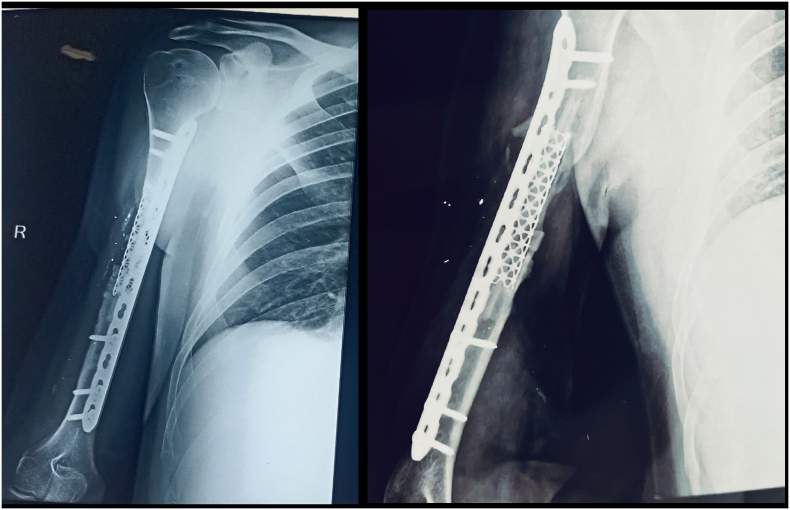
Anteroposterior (AP) and lateral radiographs of the right arm at 2-year postoperative follow-up depicts integration of bone graft with solid union around the cage.
